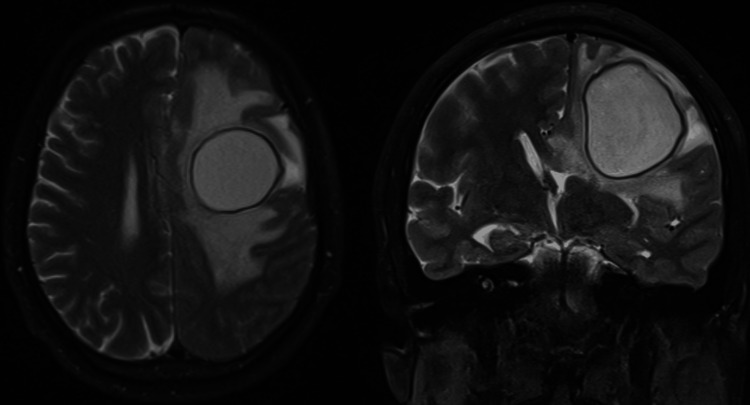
T2 MRI showing the hyperintense center, hypointense capsule, and vasogenic edema surrounding the abscess
